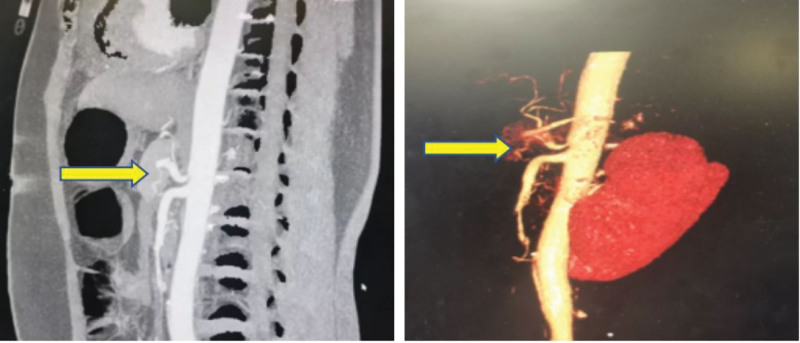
Contrast-enhanced CT: coronal reconstruction of celiac trunk compression (yellow arrow). CT = computed tomography.
